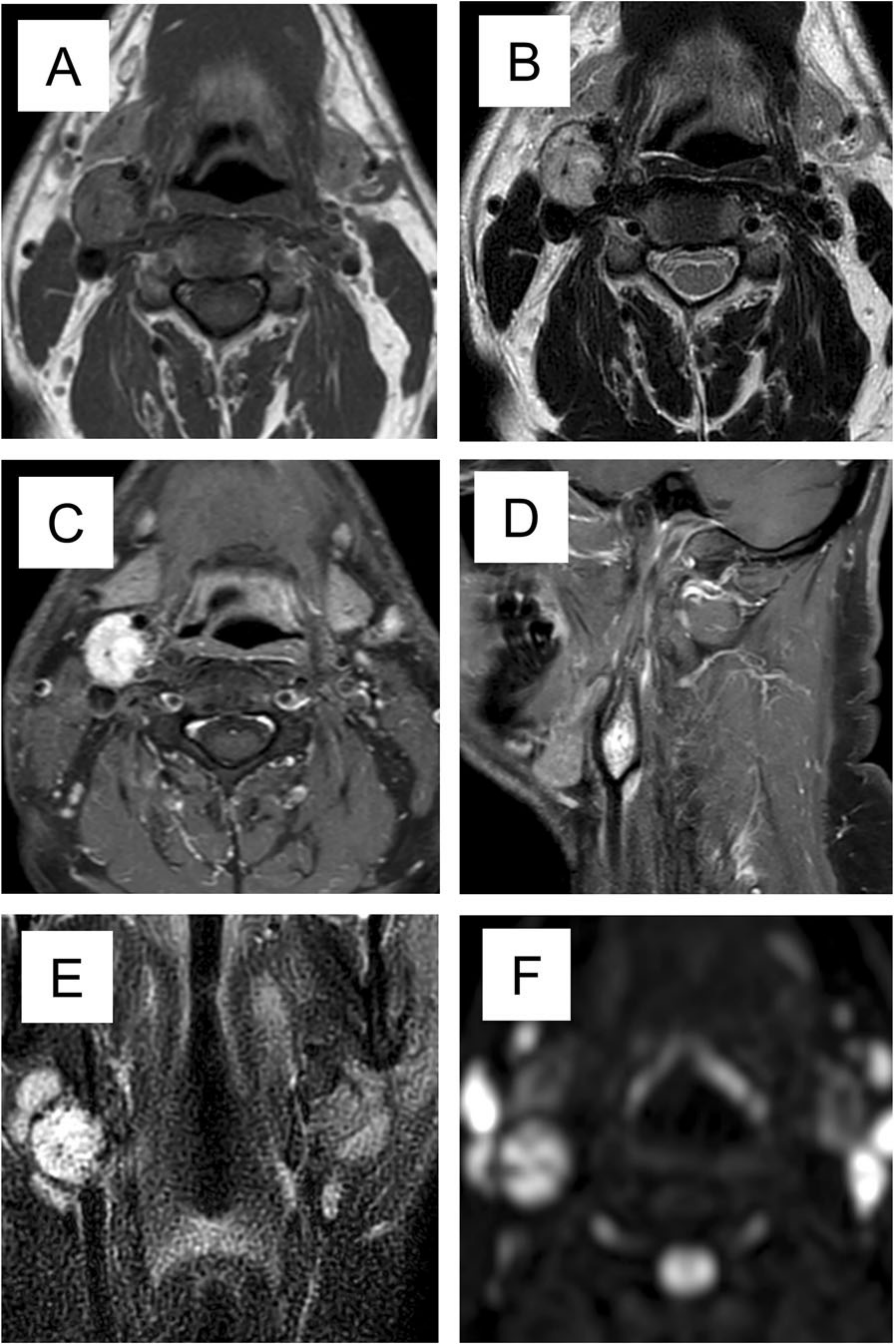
Neck magnetic resonance imaging. a Axial T1-weighted sequence; b axial T2-weighted image; c, d axial and sagittal images after contrast enhancement; e coronal short tau inversion recovery sequence; f diffusion-weighted sequence
